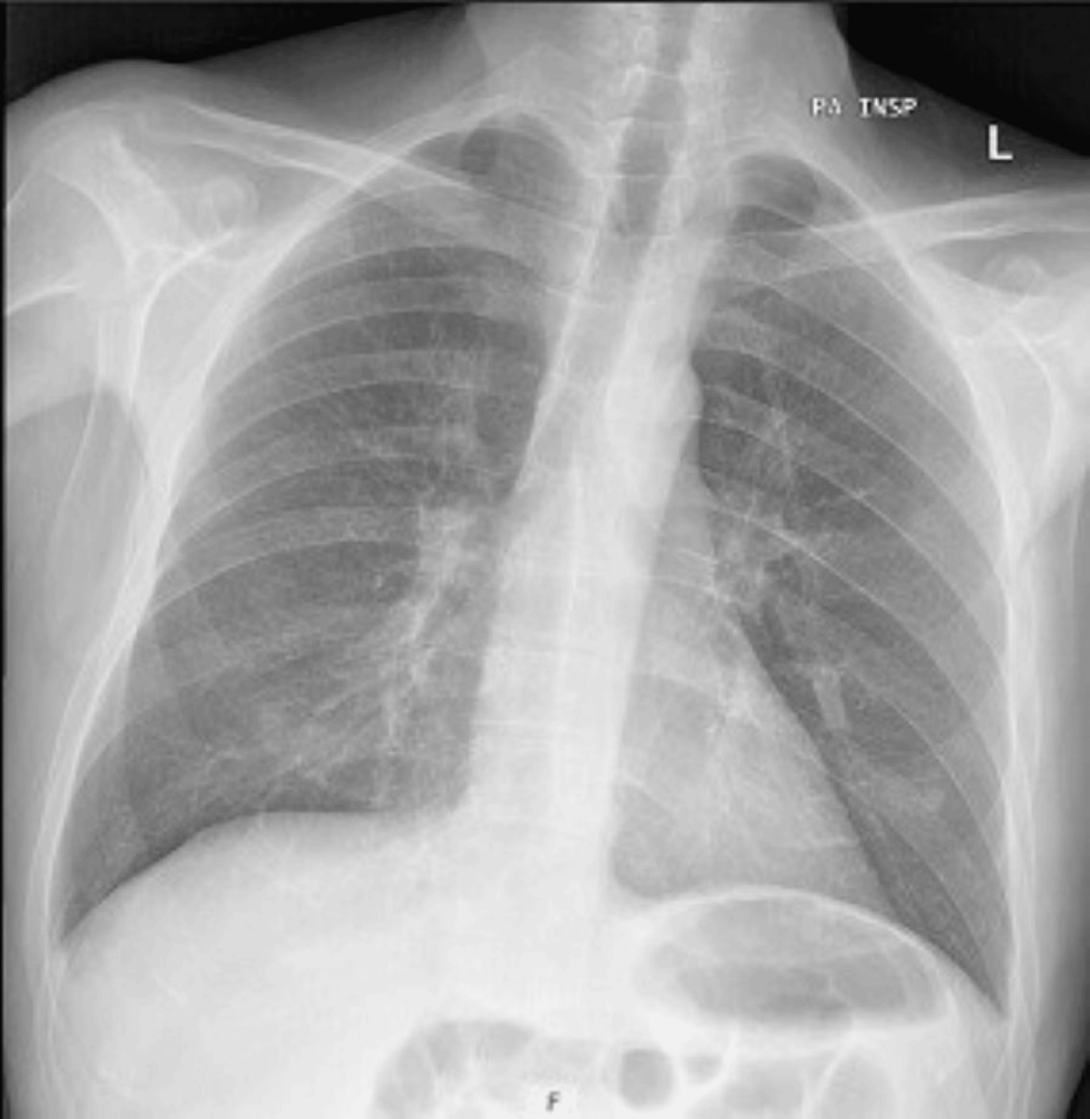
Chest X-ray without significant alterations
